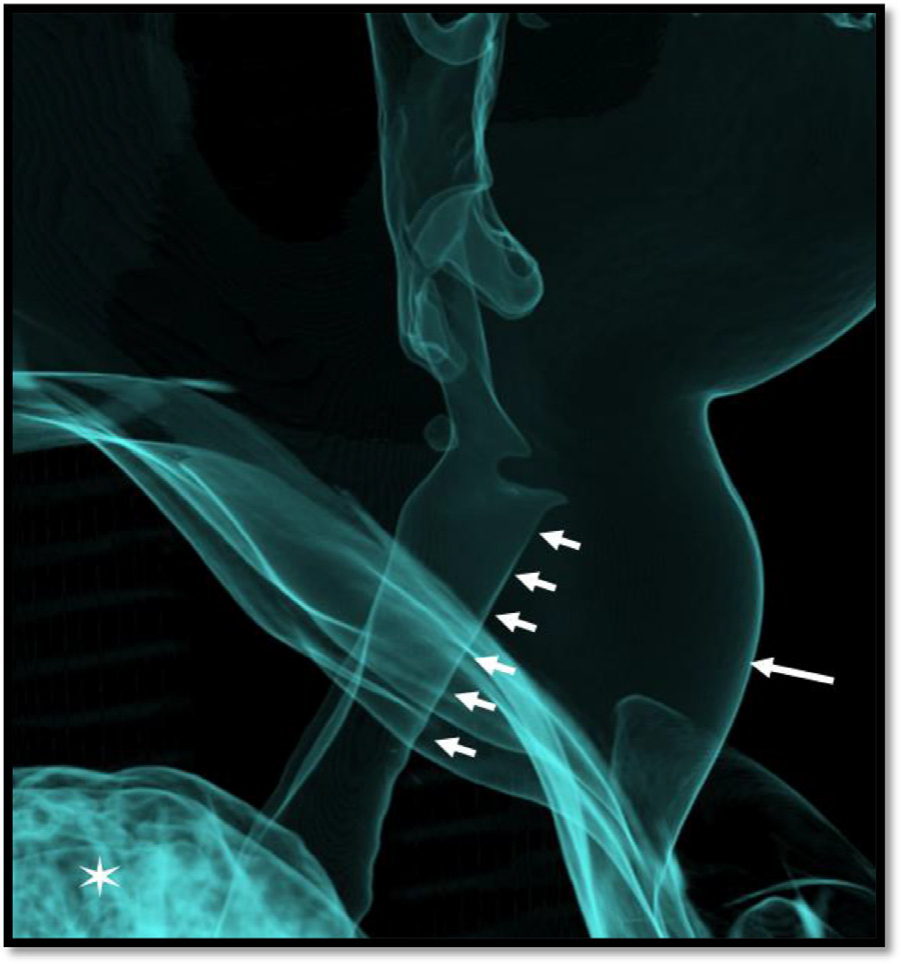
3D CT volume rendered images for air spaces showing no obvious compression effect by the colloid cyst on airway (multiple small arrows). The surface bulge of the neck swelling (long arrow). Air seen in the lung apices (asterisk).
